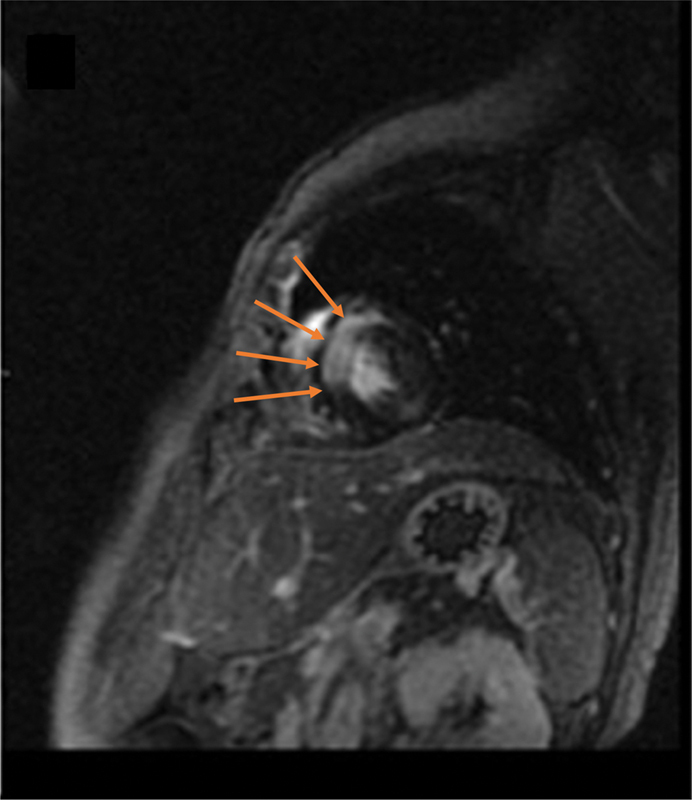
Cardiac magnetic resonance imaging demonstrates late gadolinium mural enhancement of the interventricular septum (
